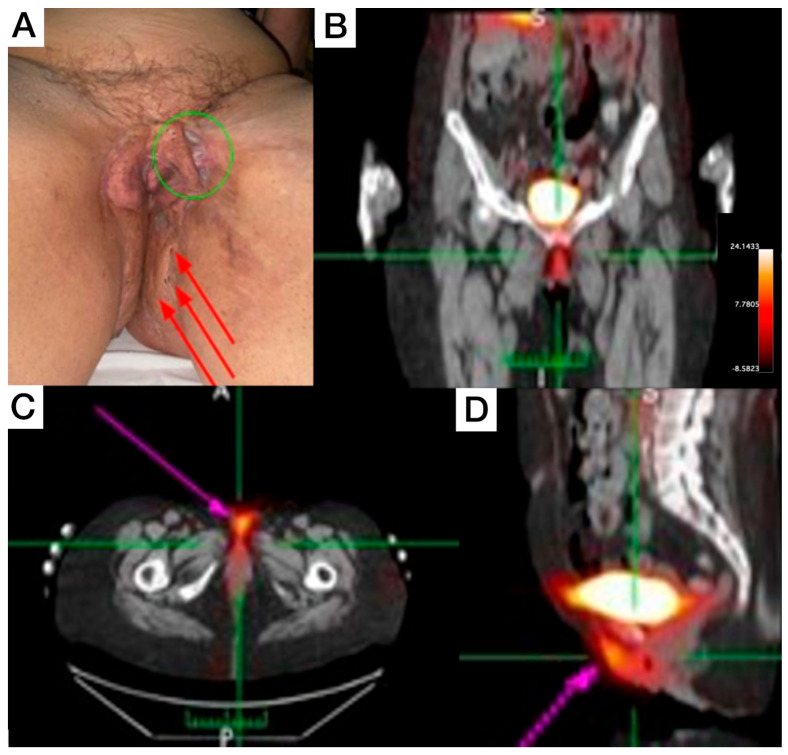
Patient 2 clinical (A) and SPECT/CT imaging findings (B–D). (A) Displays an extensive lesion affecting the vulva and perineum, presenting simultaneously in various evolutionary stages, including comedones, abscesses, and inactive cicatricial lesions (red arrows). On the anterolateral aspect of the left thigh root, an active lesion is visible, characterized by the presence of abscesses and a violaceous discoloration (green circle). This area also exhibits cicatricial tissue from a surgical anastomosis, which extends to the contralateral labium majus. (B–D) [^99m^Tc]Tc-anti-TNF-alpha scintigraphy SPECT/CT images show abnormal uptake in the genital and perineal regions (pink arrows).
